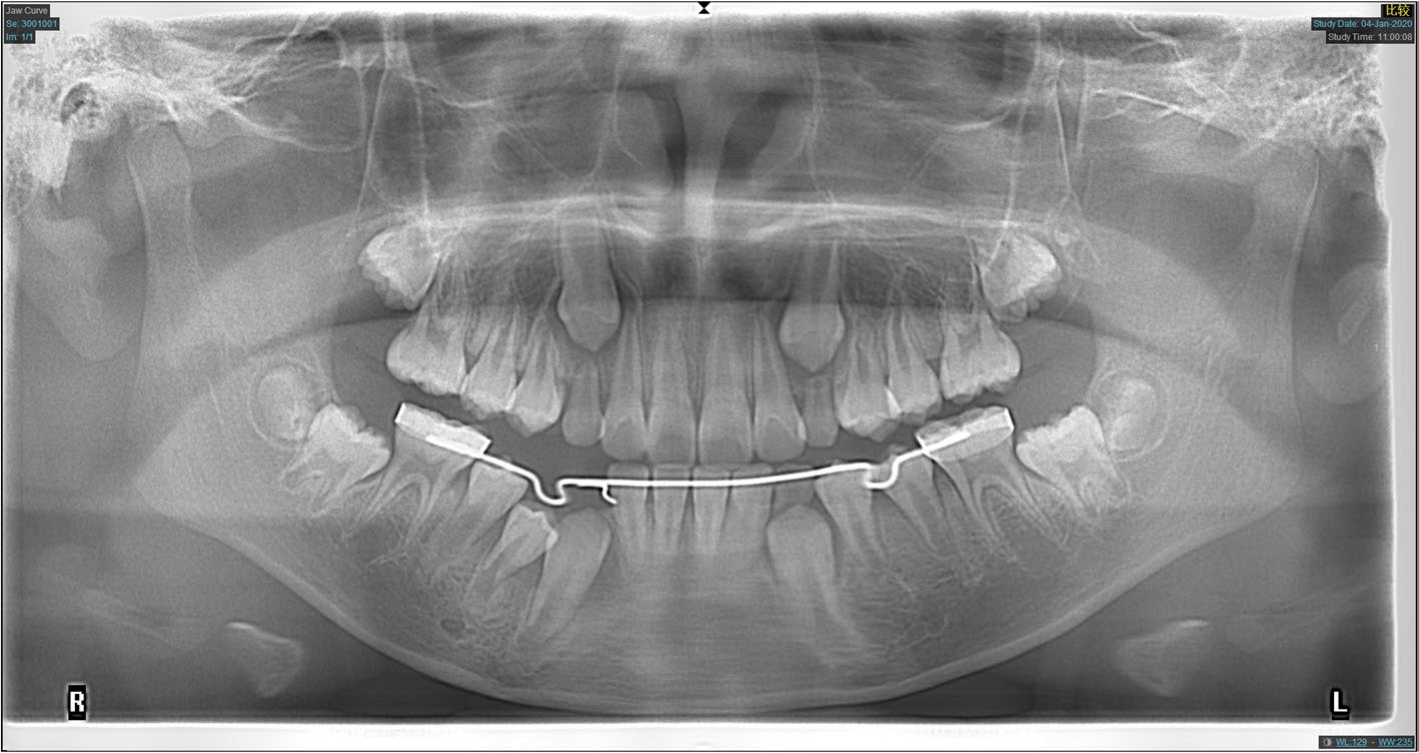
Panoramic radiograph of the patient shown in Fig. 2 after 6 months of decompression
